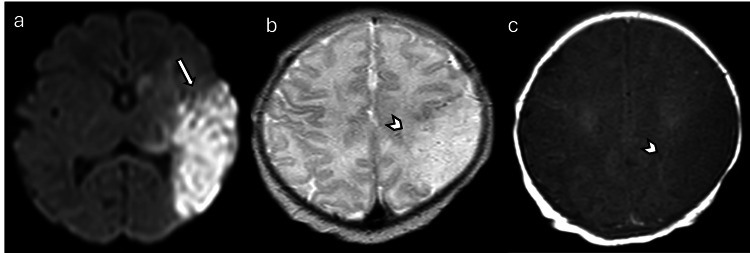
Perinatal stroke in a two-day-old patient with seizures (a) Axial DWI shows an area of restricted diffusion involving the left MCA distribution, including the left temporal lobe and watershed zones in the left parietal region and the posterior aspect of the left frontal lobe (arrow). (b) Axial T2W image demonstrates edema (arrowhead), and (c) axial T1W image shows loss of the normal T1 hyperintensity in the left peri-Rolandic area (arrowhead). Images obtained by the authors from the institutional PACS. All images are de-identified and used in accordance with institutional policy. DWI: diffusion-weighted imaging; MCA: middle cerebral artery; T2W: T2-weighted; T1W: T1-weighted; PACS: picture archiving and communication system
